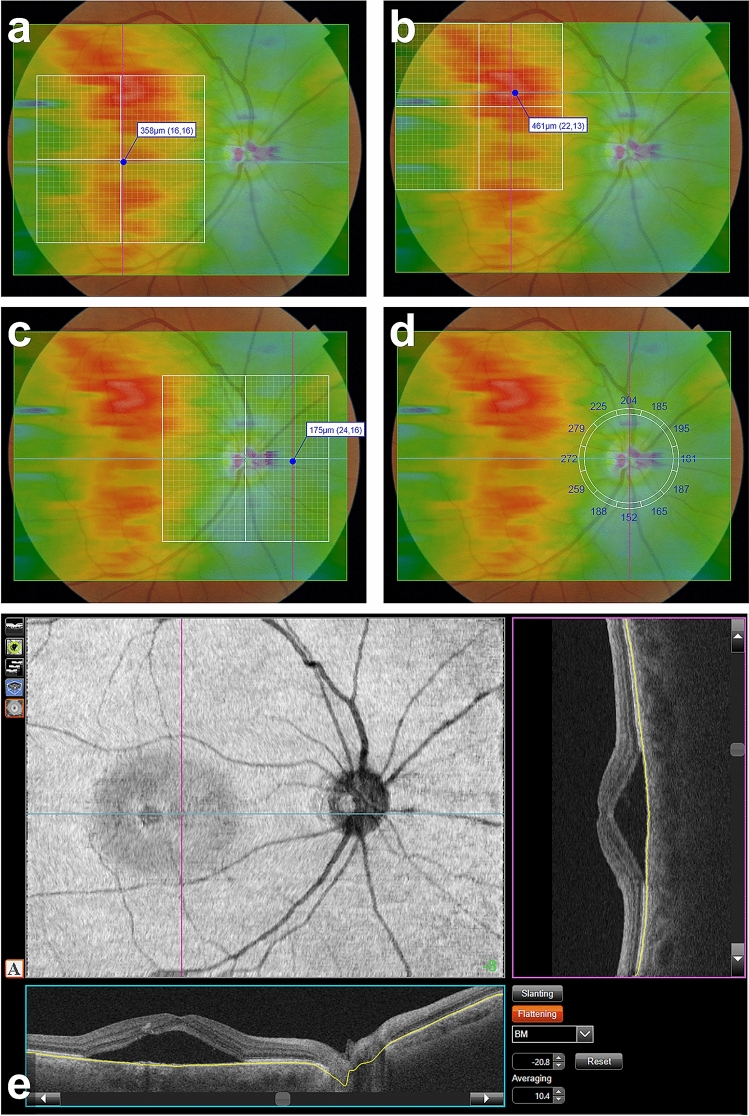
Measurement of choroidal thickness (CT) and determination of choroidal and retinal geometric landmarks. Each CT was measured using a color-coded CT map and a SuperPixel-200 grid on swept-source optical coherence tomography (SS-OCT). (a) Subfoveal choroidal thickness (SCT), (b) greatest choroidal thickness (GCT), and (c) nasal peripapillary choroidal thickness (NPCT) were measured semi-automatically after the superimposition of a color-coded CT map and a SuperPixel-200 grid on the color fundus photograph using the manufacturer’s software. (d) The location of NPCT measurement was determined using a 3.4-mm diameter circular grid for retinal nerve fiber layer analysis. The locations of the three reference geometric landmarks – the foveal centre, optic disc centre, and GCT point – were determined on en face SS-OCT images using the built-in software (d and e). The foveal centre and optic disc centre were marked on the en face image at the corresponding point where the two vertical lines intersected. The x and y coordinates of the GCT point was determined during the GCT measurement using a SuperPixel-200 grid (b).
